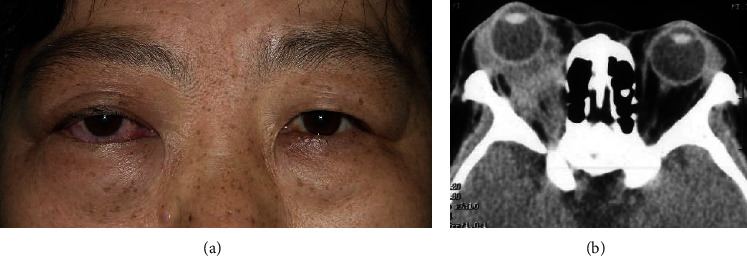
(a) Clinical photograph of a 49-year-old woman with orbital metastasis of breast cancer showed proptosis, ptosis, and chemosis of the right eye. (b) The coronal computed tomography showed an irregular mass in the right orbit, surrounding the eyeball, exophthalmos, with extraocular muscle involvement.
